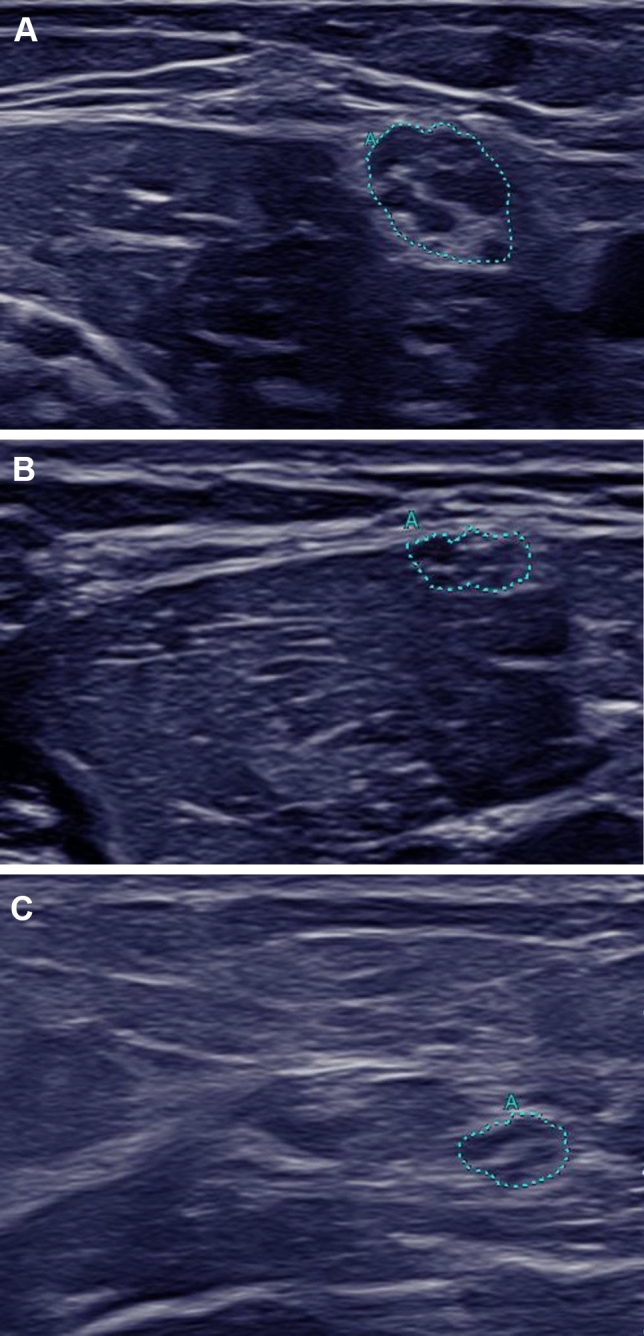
Nerve ultrasound of the right ulnar nerve measured at the upper arm
